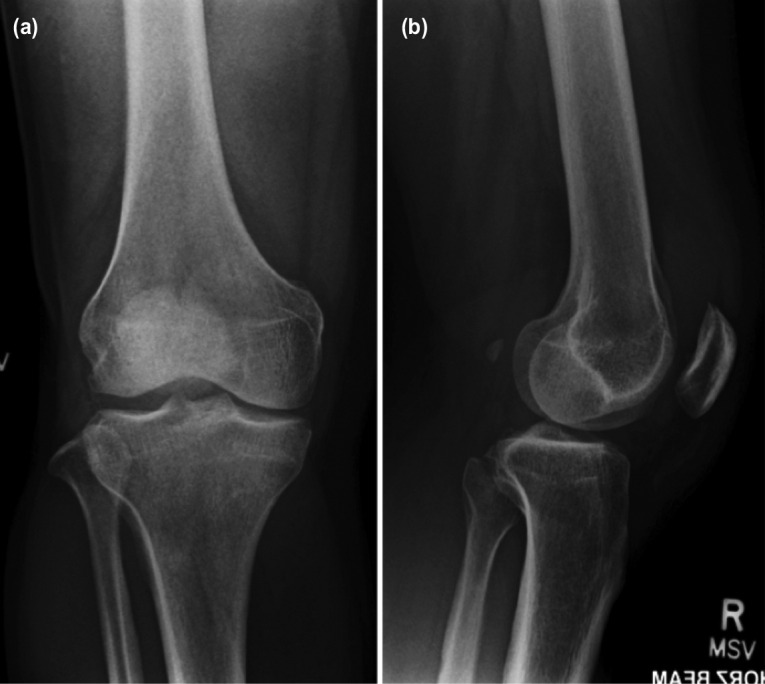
(a) Antero-posterior and (b) lateral radiographs of the right knee did not reveal any radiographic signs of chondrocalcinosis.
